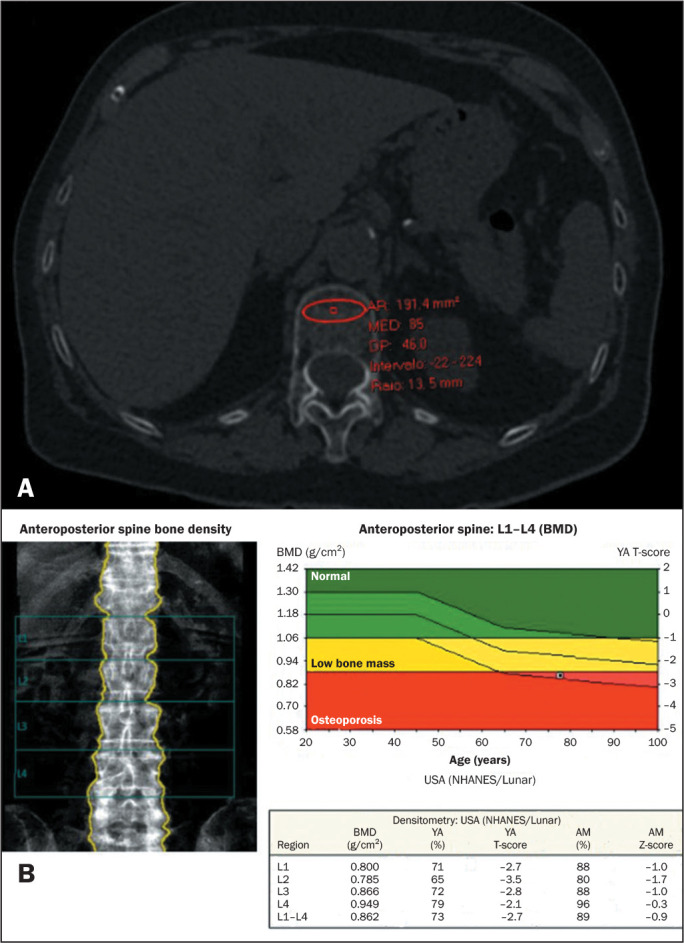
A: Abdominal CT of a 69-year-old woman, performed to investigate
